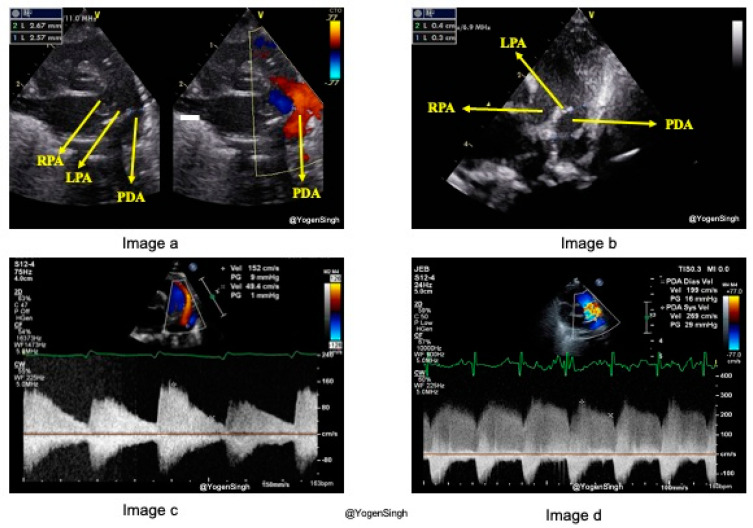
Characteristics of patent ductus arteriosus (PDA). (a) shows the measurement of PDA simultaneously on 2D and color flow mapping. (b) shows the measurement of a tortuous PDA in 2D; the narrowest part should be reported. (c) shows the pulsatile flow pattern on Doppler assessment, and (d) shows a constricting flow pattern. *LPA—left pulmonary artery, RPA—right pulmonary artery, PDA—patent ductus arteriosus*.
